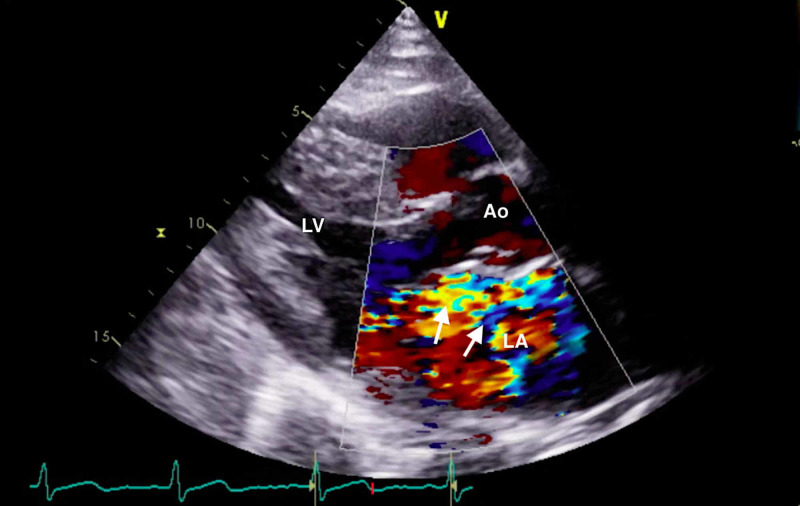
Comprehensive transthoracic echocardiogram (parasternal long-axis window) with color Doppler demonstrating flail motion of the posterior mitral leaflet and a severe eccentric mitral regurgitant jet (arrows). Ao, aortic root; LA, left atrium; LV, left ventricle.
